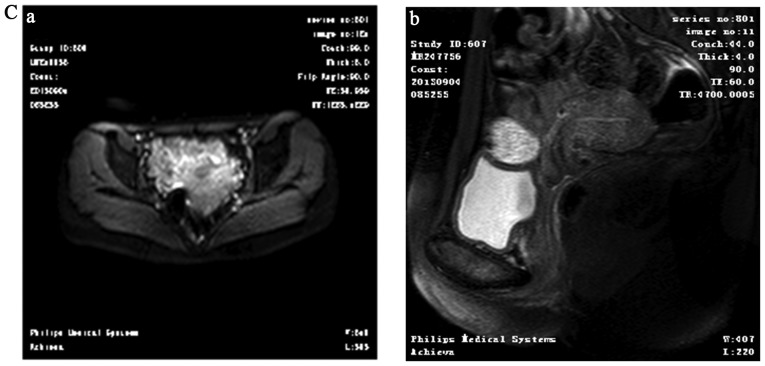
MRI of the pelvis. (A) Prior to treatment, MRI identified an ~6.0×6.0-cm cervical carcinoma, and invasion of the posterior wall of vagina and pelvic lymph nodes. (B) Following two cycles of NACT for six weeks, a decrease in the volume of the vaginal mass was observed. (C) Following four cycles of NACT for 12 weeks, complete clinical remission was achieved. (a) transverse section and (b) median sagittal section. MRI, magnetic resonance imaging; NACT, neoadjuvant chemotherapy.
